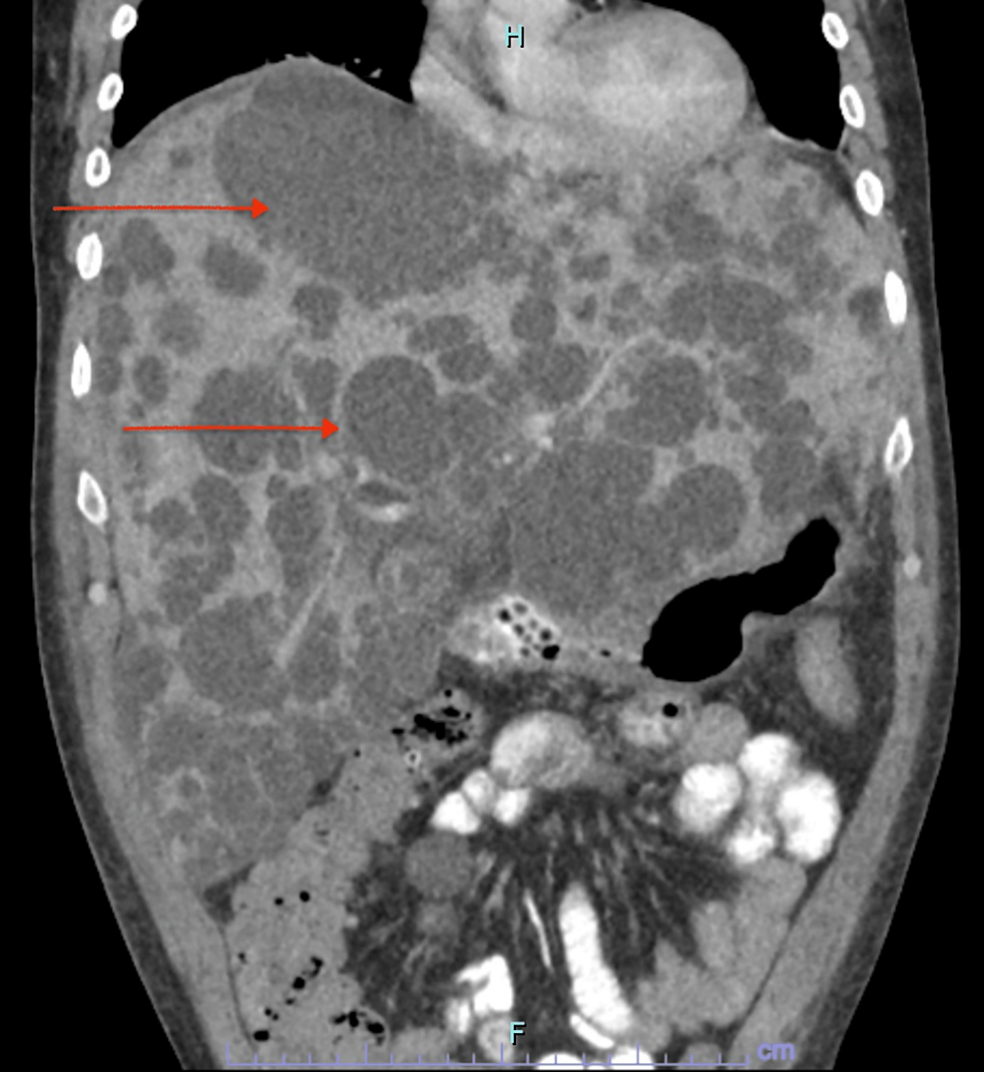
Computed tomography of the abdomen and pelvis without contrast showing liver cysts (arrows)
